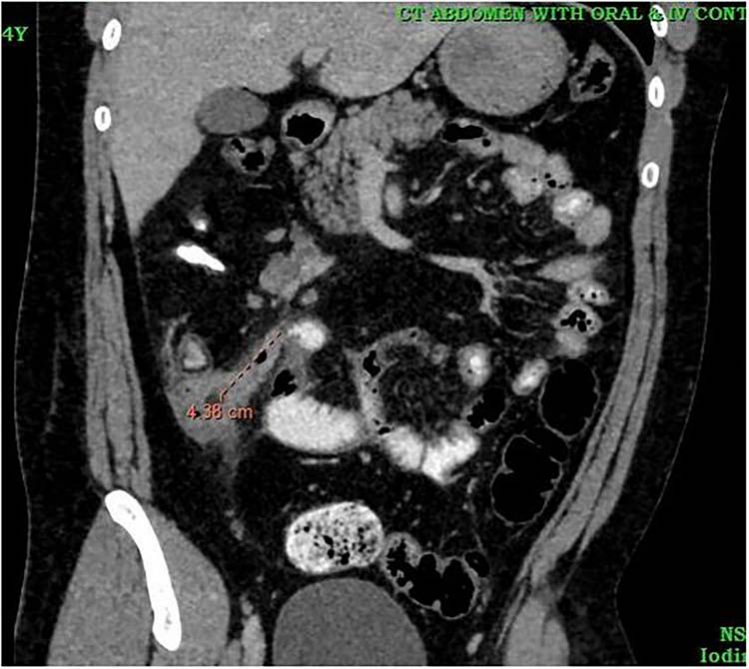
CT image showing inflammed stump appendix.
